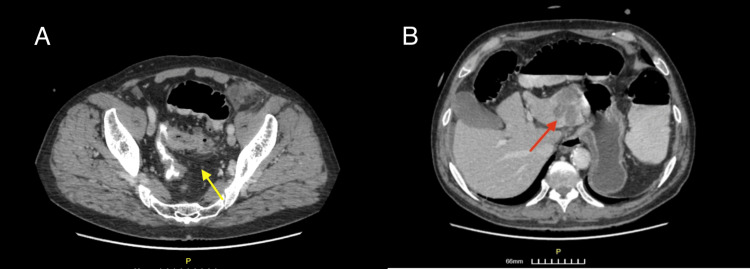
Contrast-enhanced CT after per os and IV contrast administration. Axial images. A. Bowel obstruction due to colonic neoplasia (yellow arrow), with segmental thickening and presence of diverticula. B. Hepatic metastatic lesion (red arrow). Air-fluid levels are also evident.
